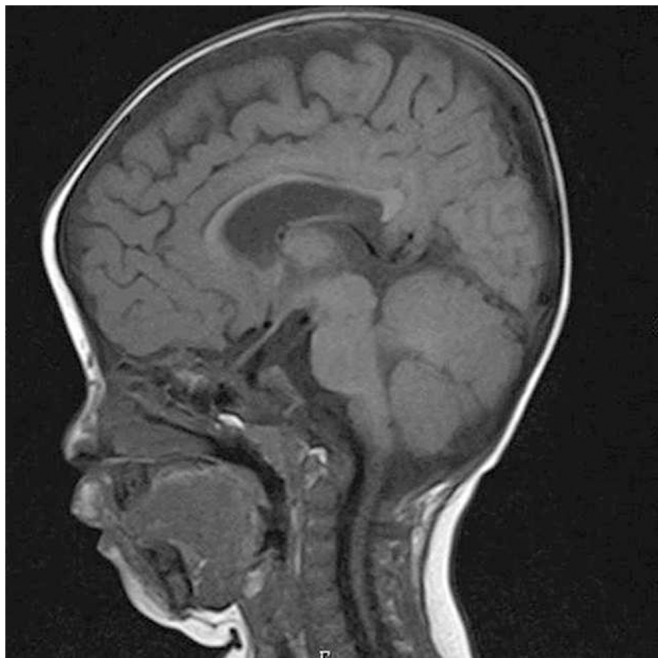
Cranial MRI findings at 10 months of age. Sagittal and T1-weighted image, showing mild hypoplasia in the corpus callosum.
